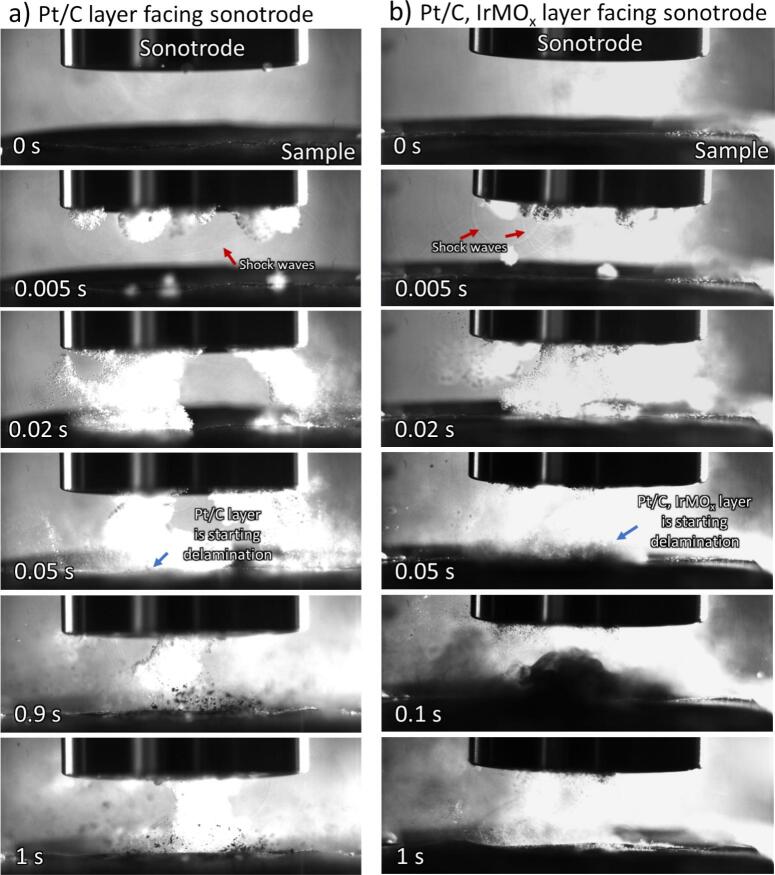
Images showing snapshots taken from a high-speed camera (20 k frames per s) during ultrasonic delamination of the fuel cell sample in water: (a) Pt/C cathode side facing sonotrode; (b) Pt/C, IrMO_x_ anode side facing sonotrode. The sonotrode was 20 mm in diameter, with 40 W cm^−2^ power intensity, at 5 mm from the substrate.
